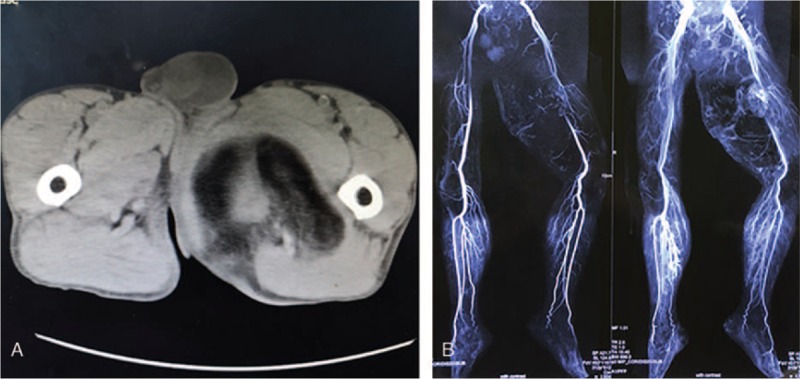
Preoperative CT and MRA findings. CT showed a very low-density shadow in the soft tissue inside the left thigh, showing a large amount of fat density (A). There were no significant abnormalities found on the MRA of the lower extremity vessels (B). CT = computed tomography, MRA = magnetic resonance angiography.
